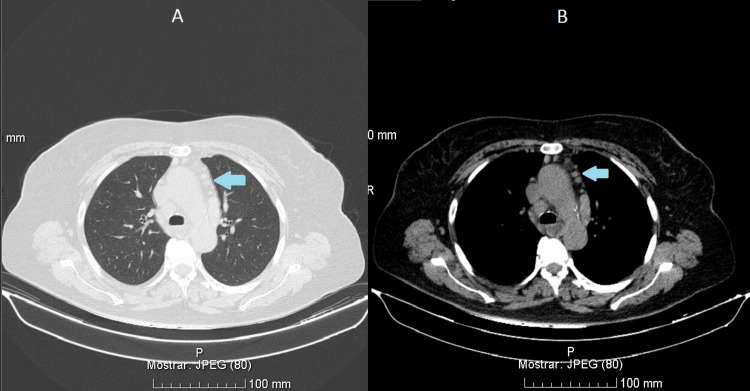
Chest computed tomography demonstrated a solitary, well-defined 7 × 5 mm pulmonary nodule in the left upper lobe, along with multiple anterior mediastinal lymphadenopathies (A: lung window; B: mediastinal window)
